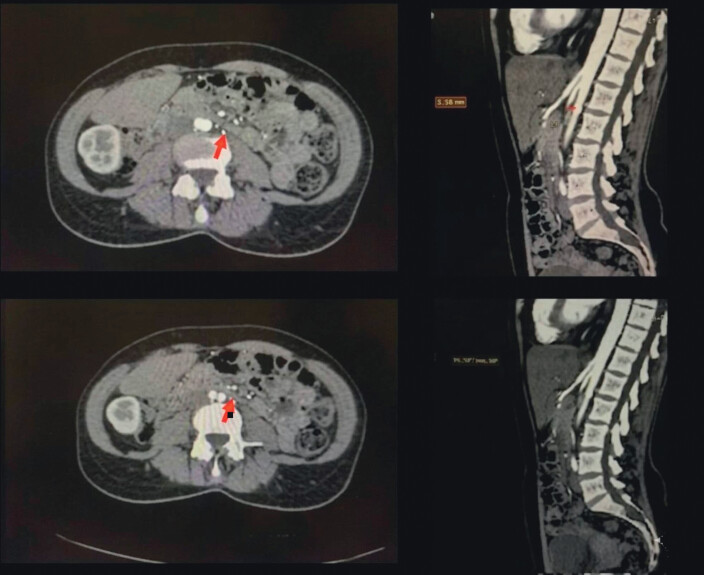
CT angiography confirming an aortomesenteric angle of 14.7° and an aortomesenteric distance of 5.36 mm, with an absence of mesenteric fat, diagnostic of superior mesenteric artery syndrome.
